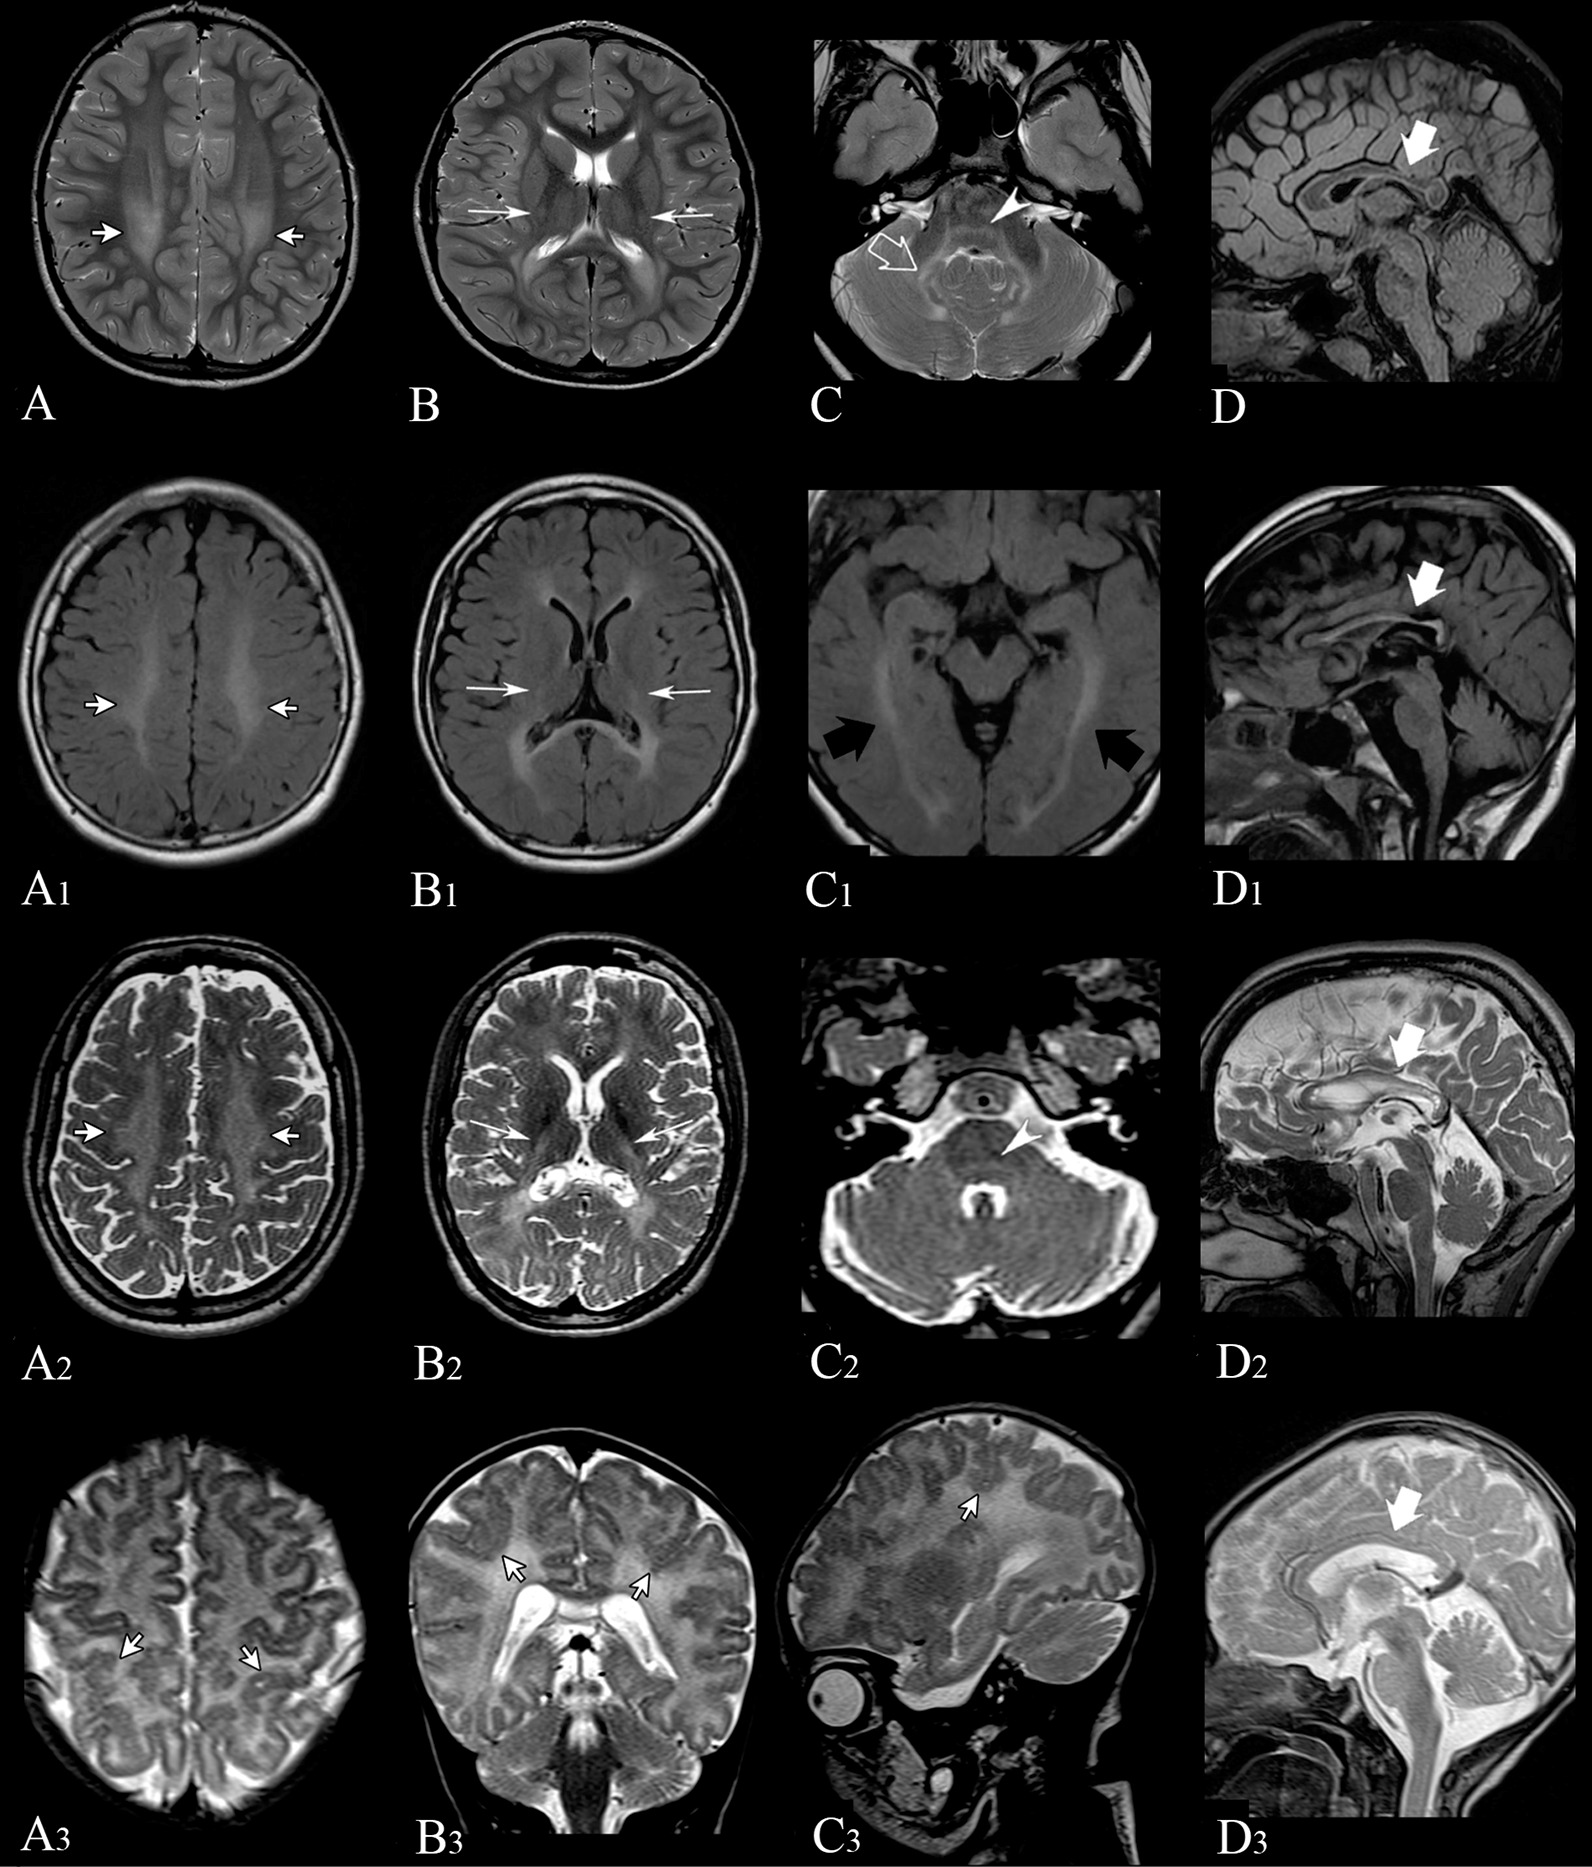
Brain MRI images of individual A.II-3 (A–D) at 8 years of age. Axial (A–C) T2-weighted images show bilateral hyperintensity within the posterior periventricular white matter (short white arrows in A), posterior limb of the internal capsules (long white arrows in B), within the cerebellar peduncles and dentate nuclei in the cerebellar region (empty white arrow in C) and in medial lemnisci (white arrowhead in C). Sagittal FLAIR image (D) shows thinning of the posterior portions of the corpus callosum (white arrow). Brain MRI images of individual C.II-3 (A1–D1) at 11 years of age. Axial (A1–C1) FLAIR images show bilateral hyperintensity within the posterior periventricular white matter (short white arrows in A1), posterior limb of the internal capsules (long white arrows in B1), and optic radiations (black arrows in C1). Sagittal FLAIR image (D1) shows thinning of the corpus callosum (white arrow). Brain MRI images of individual C.II-2 (A2–A2) at 16 years of age. Axial (A2–C2) T2-weighted images show bilateral hyperintensity within the posterior periventricular white matter (short white arrows in A2), posterior limb of the internal capsules (long white arrows in B2), and in medial lemnisci (white arrowhead in C2). Sagittal T2-weighted image (D2) shows thinning of the corpus callosum (white arrow). Brain MRI images of Individual D.II-3 (A3–D3) at 1 month of age. Axial (A3), Coronal (B3), and Sagittal (C3) T2-weighted images show bilateral malformation of cortical development in parietal lobes, with a polymicrogyria-like appearance (short white arrows). Midline Sagittal T2-weighted image (D3) does not show thinning of the corpus callosum at this early stage of life (white arrow)
